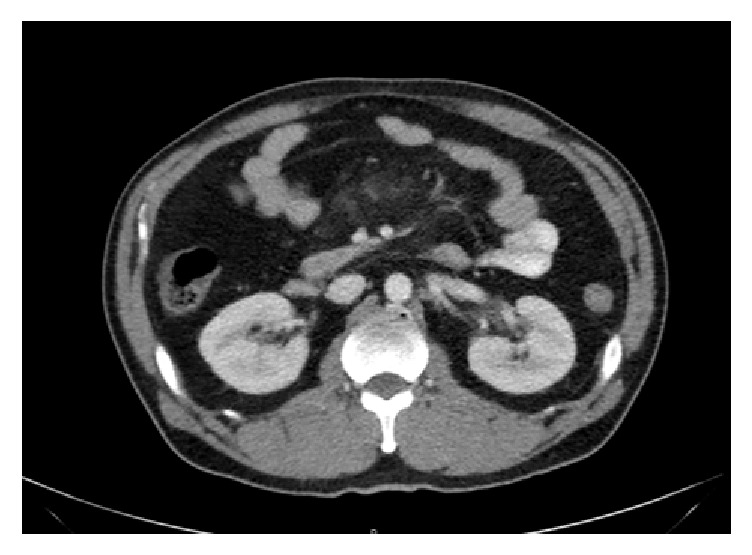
CT scan of the abdomen and pelvis showing inflammatory fat stranding in the small bowel mesentery (axial view).
